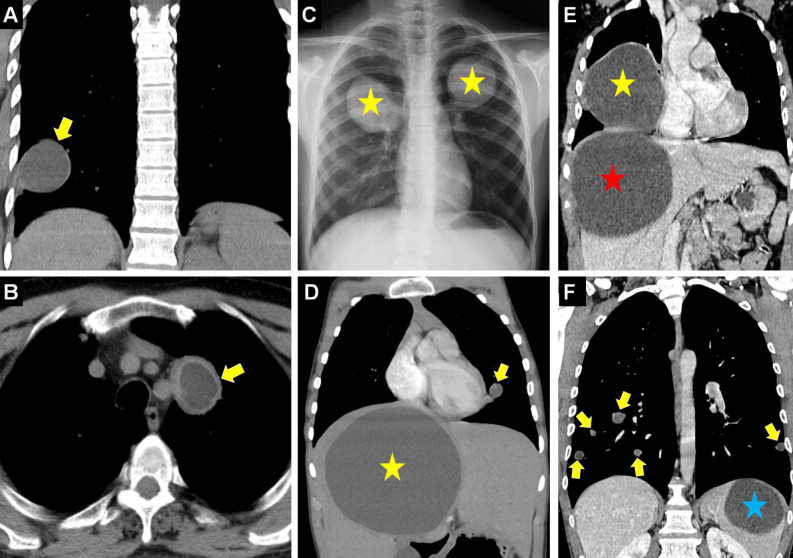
Coronal compued tomography (CT) (A) shows a thin-walled, unilocular, low-density, and uncomplicated hydatid cyst (HC) (arrow). Axial CT (B) shows a unilocular hydatid cyst appearance with a thickened wall structure (arrow). Chest radiograph (C) shows bilateral pulmonary HCs (asterisks). Coronal CT (D) shows simultaneous multiorgan involvement with HCs in the liver (asterisk) and left lung (arrow). Coronal CT (E) shows giant HCs in the liver dome (red-asterisk) and right lung (yellow-asterisk). Coronal CT (F) shows simultaneous HCs in the spleen (asterisk) and bilateral lungs (arrows).
